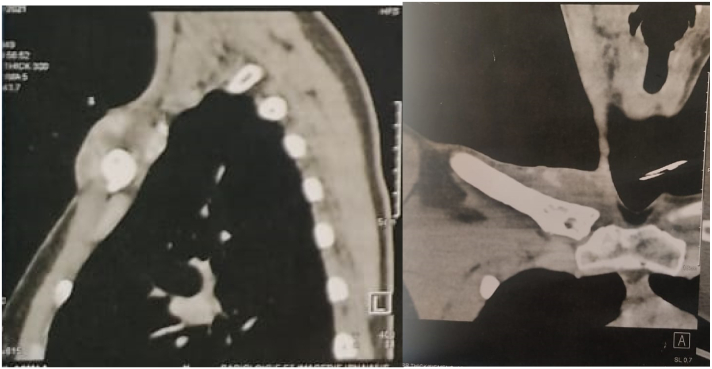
Post-chemotherapy CT scan showing type condensation of the clavicle and sternal manubrium.
